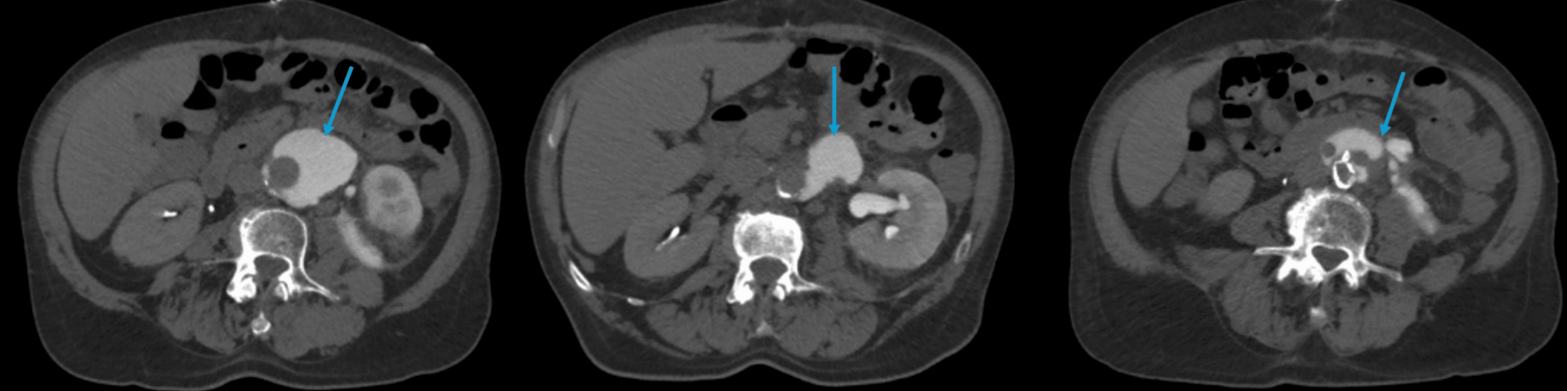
Axial CT urogram (delayed phase) showing contrast extravasation from the left ureter into the retroperitoneal collection (blue arrows), consistent with urinoma formation adjacent to the vascular graft.
